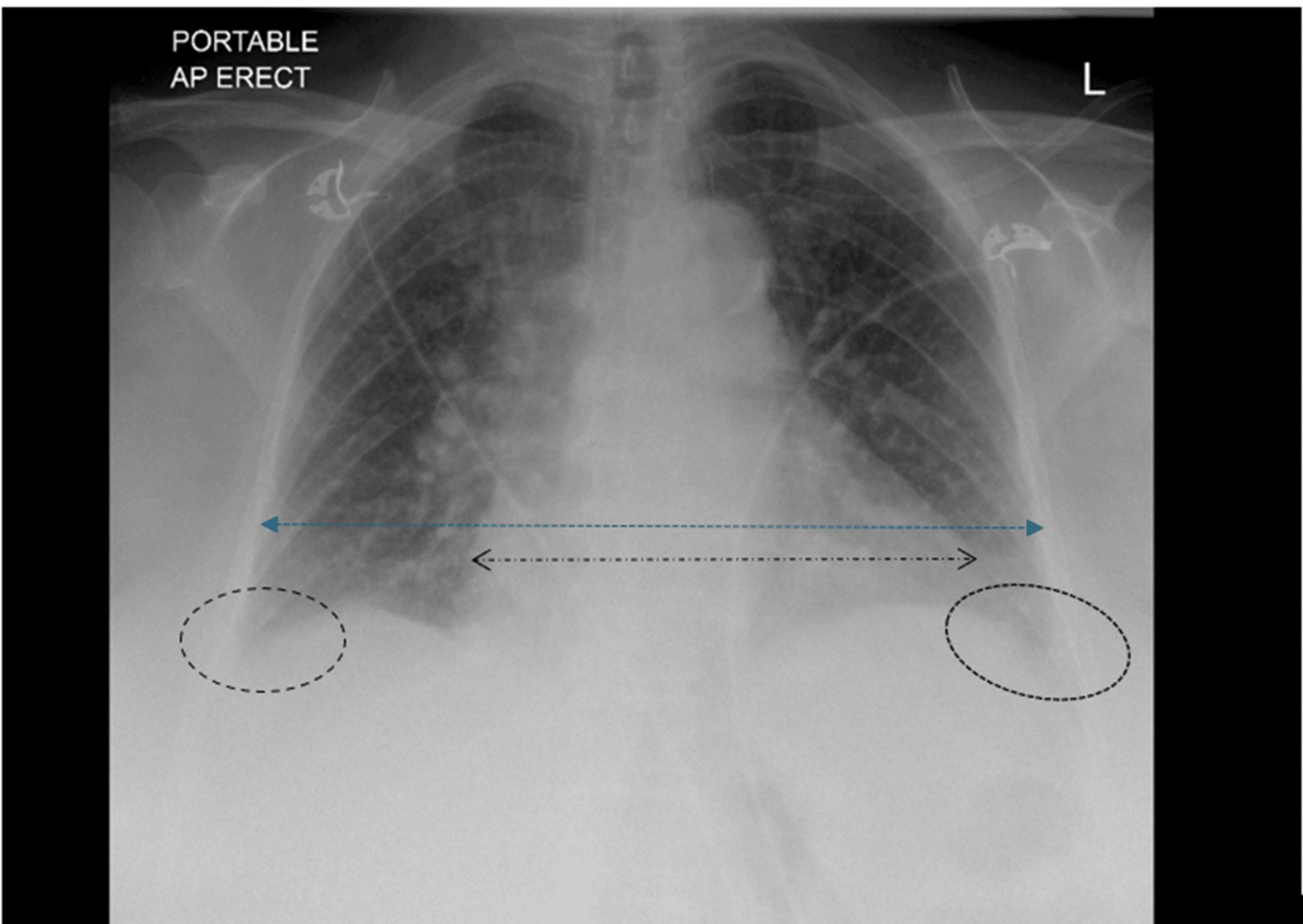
Chest X-ray In this image, the heart borders have been highlighted in comparison to the thoracic borders, which clearly demonstrates a cardiothoracic ratio of >0.5. The costophrenic angles are also blunted, which have been highlighted bilaterally. As can be seen in this patient's chest X-ray, an increased cardiothoracic ratio and blunting of the costophrenic angles are visible, suggesting heart failure with pleural effusion, potentially secondary to heart failure.
